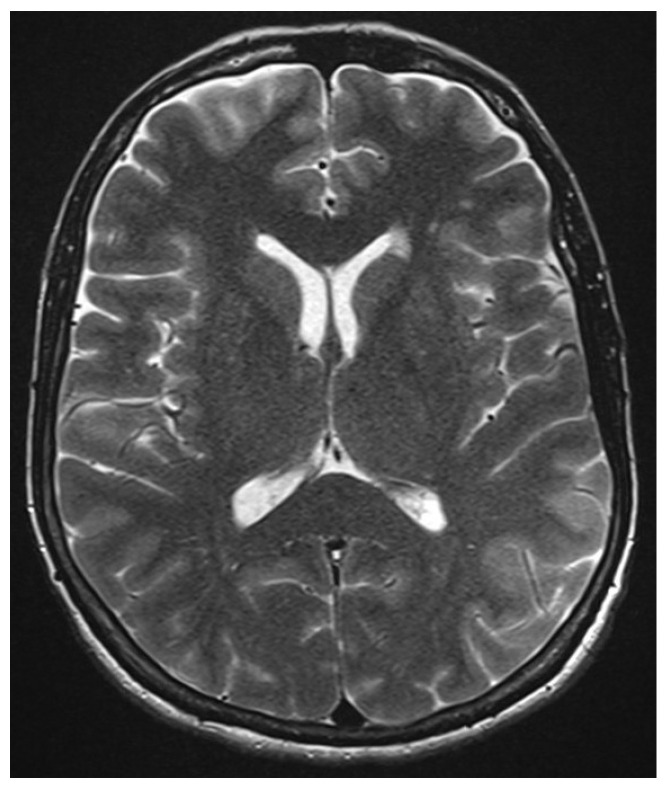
Neuroimaging. Cranial magnetic resonance imaging (cMRI) showed mild cerebral microangiopathy and nonspecific gliosis in the subcortex.
